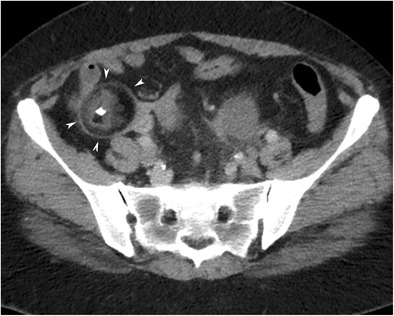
Axial CT image of an 18-year-old female with mature cystic teratoma shows a tooth-like high-density structure in the centre of a Rokitansky nodule (arrowheads)
